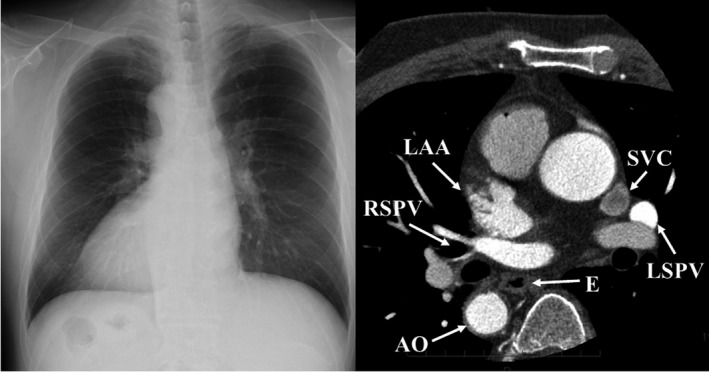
Chest X‐ray and computed tomography showing dextrocardia with complete situs inversus without any other anatomical complications. AO, aorta; E, esophagus; LAA, left atrial appendage; LSPV, left superior pulmonary vein; RSPV, right superior pulmonary vein; SVC, superior vena cava
